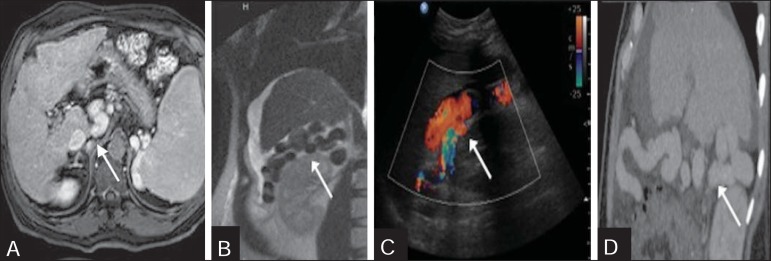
A: Contrast-enhanced, T1-weighted magnetic resonance imaging
